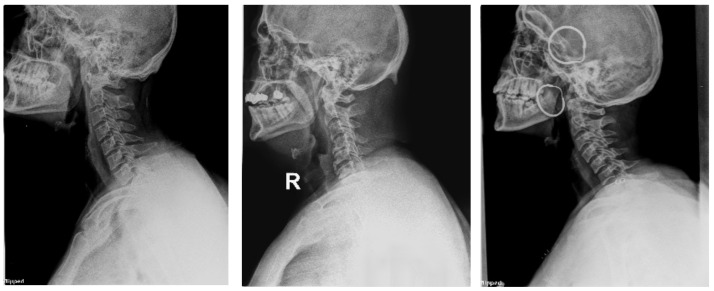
X-ray images for the normal CS.
